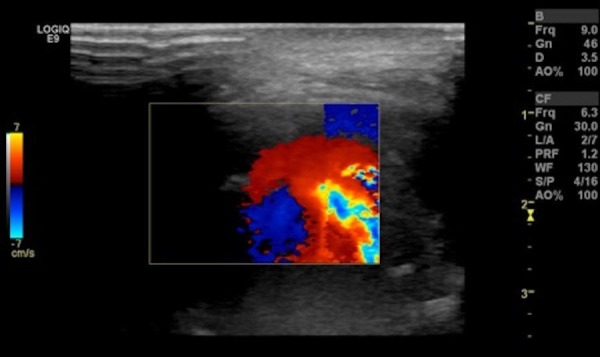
Ultrasound image of the right parotid region with Doppler interrogation reveals swirling flow within the large pseudoaneurysm in the right masticator space.
